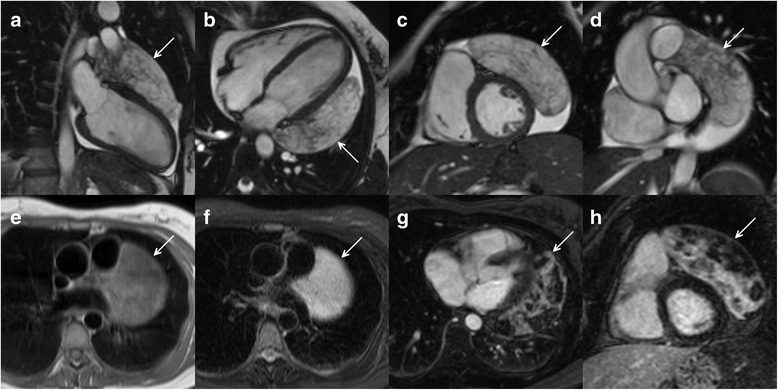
Cine movie MRI images in the long- (panel a, b) and short-axis planes in diastolic phase (panel c and d). Intermediate signal intensity on proton density-weighted images (panel e) and high signal intensity on T2-weighted SPIR images (panel f). LGE images in the long- (panel g) and short-axis planes (panel h). *Arrows* show the intrapericardial tumour
